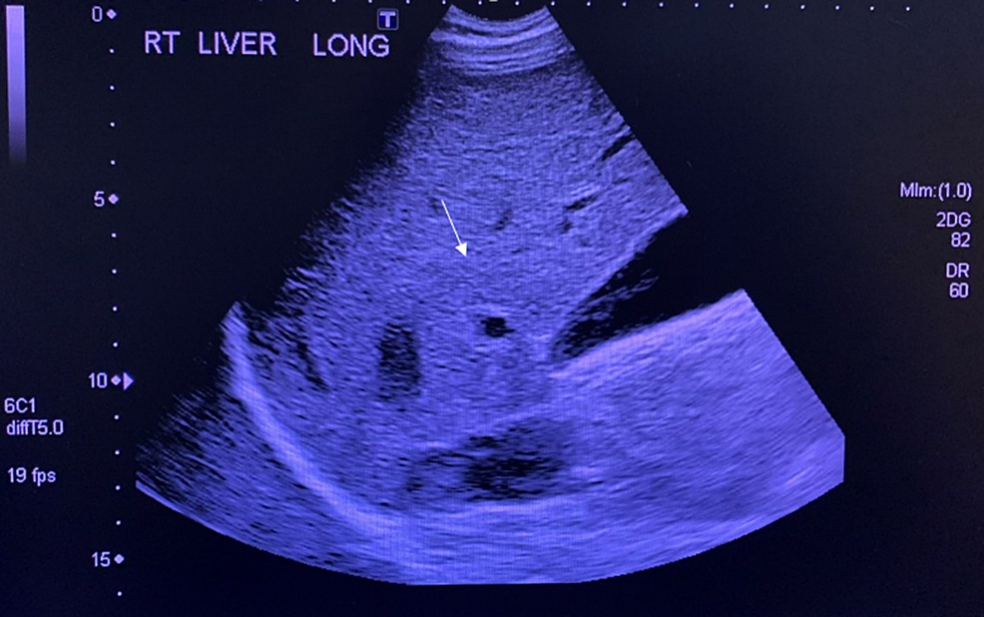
Ultrasound of the liver suggestive of fatty infiltration. Arrow pointing at a slight, diffusely increased echogenicity of a moderately enlarged liver which is a non-specific finding but can be seen in the setting of fatty infiltration.
